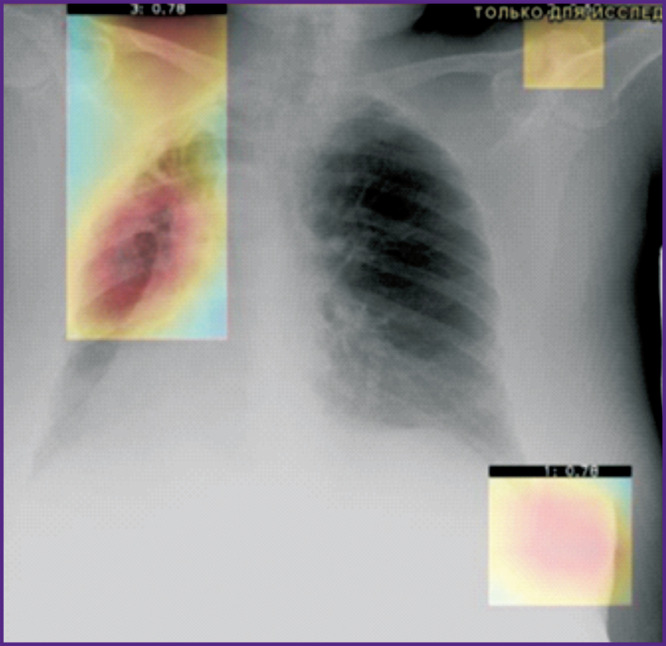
An example of an SaMD-AI technical flaw (1.3, see the Table) using evidence from chest radiography
